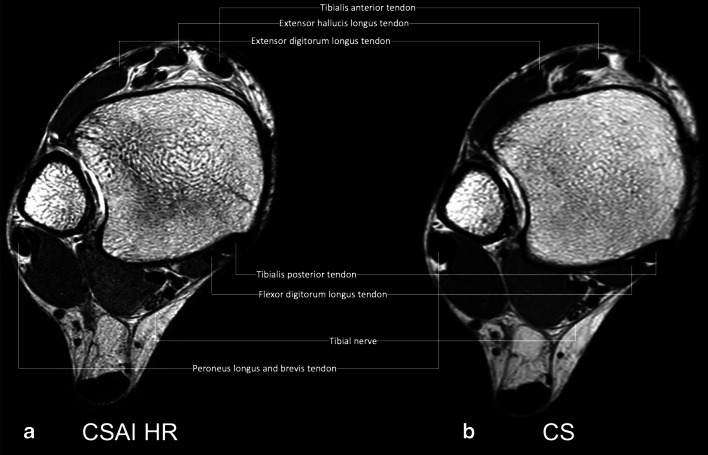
High-resolution axial T2-weighted CSAI TSE image (a, 2:50 min) and conventional CS TSE image (b, 2:25 min) of a 30-year-old female patient. Note the detailed depiction of the tendons and the tibial nerve and the improved discrimination from adjacent structures in the high-resolution CSAI TSE image compared to the CS TSE image
